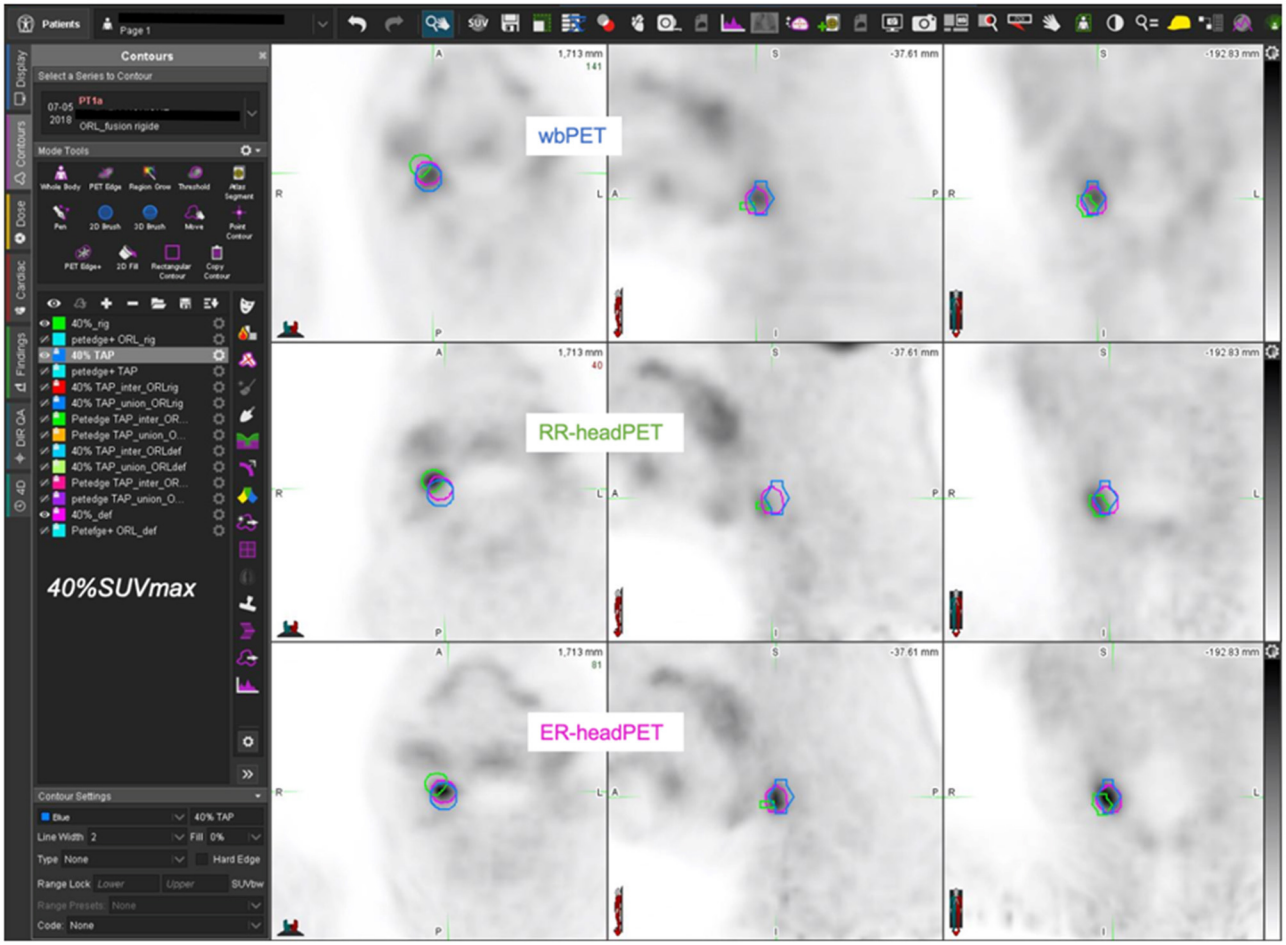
63-year-old man with T1 N1 M0 oropharyngeal SCC (AJCC stage III) – estimated MTV = 0.9cc. wbPET MTV (=V1 in blue), RR-headPET MTV (=V2 in green) and ER-headPET MTV (=V3 in pink) delineated with 40%SUVmax segmentation method reported on the wbPET (top row), RR-headPET (middle row) and ER-headPET (bottom row) images in axial (left column), sagittal (center column) and coronal (right column) views. OF (V3, V1) = 71.2% vs. OF (V2, V1) = 2.9%; DICE (V3, V1) = 74.5% vs. DICE (V2, V1) = 3.1%.
